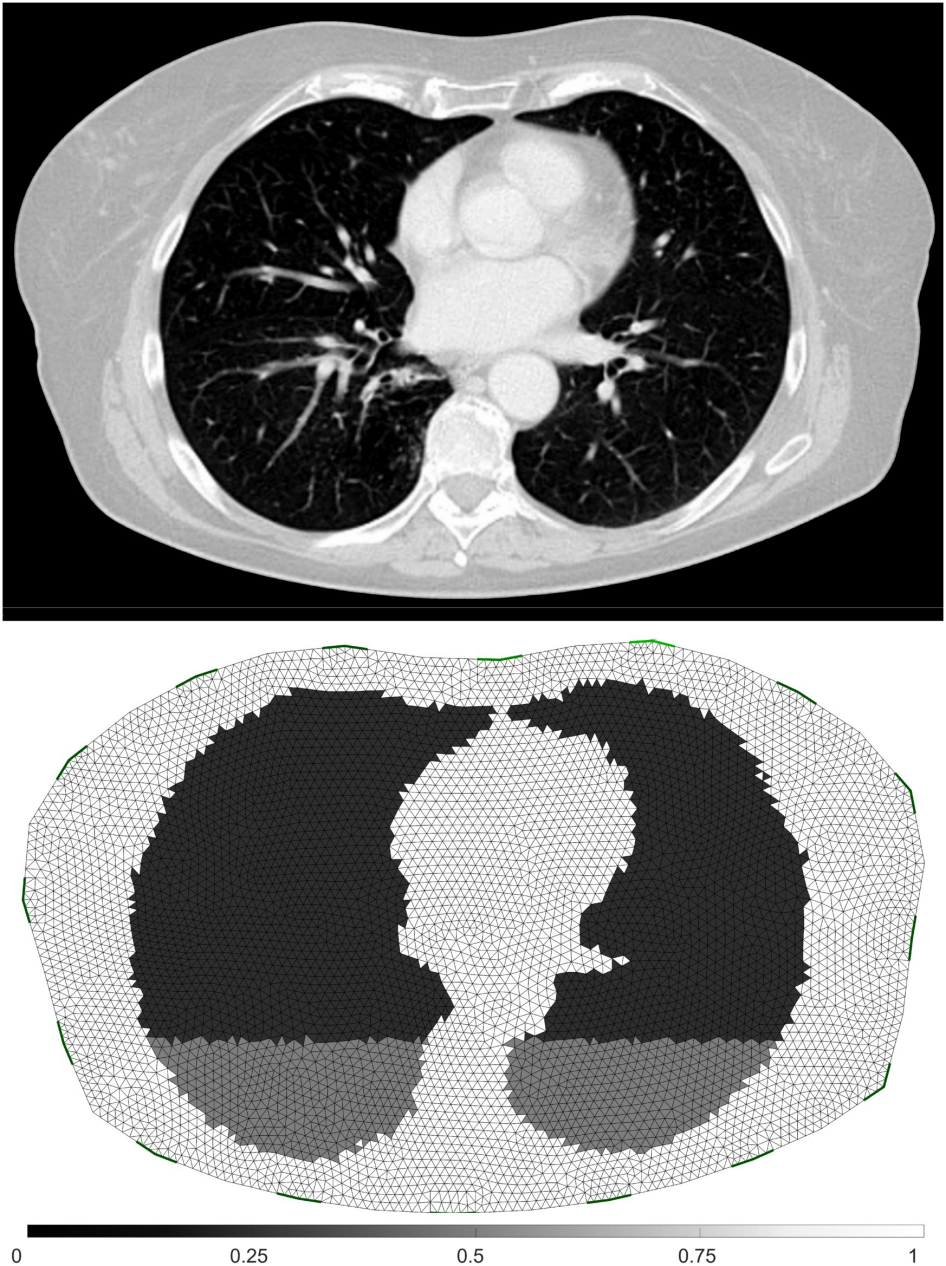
First row: The original CT image. Second row: The derived FEM model with 25% atelectasis area at the dorsal part.
